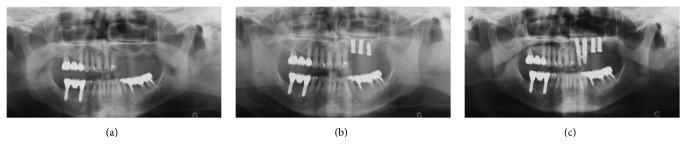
Placement of implants, panoramic radiographs. (a) Initial situation, (b) immediately after implant placement in sites 23, 24, and 25, and (c) 7 months later, immediately after implant placement in sites 25 and 26.
